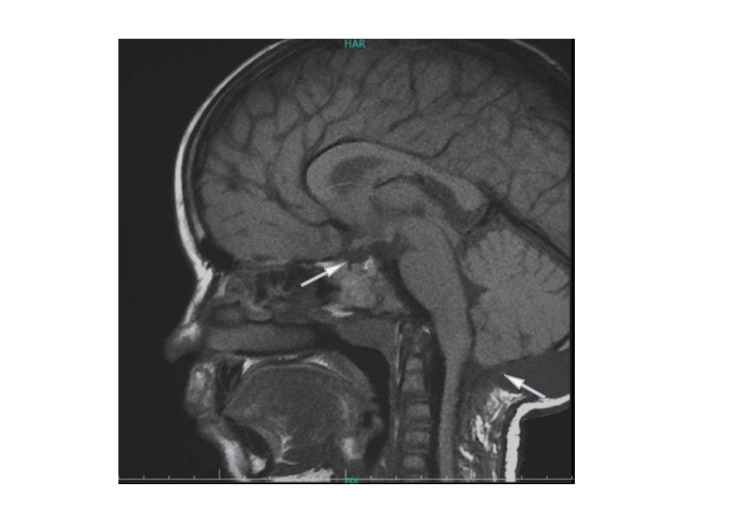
MRI of the second patient before the initiation of GH therapy, which presents slightly smaller measurements of the pituitary without any prolapse of the cerebellar tonsils. MRI: Magnetic Resonance Imaging, GH: Growth Hormone
